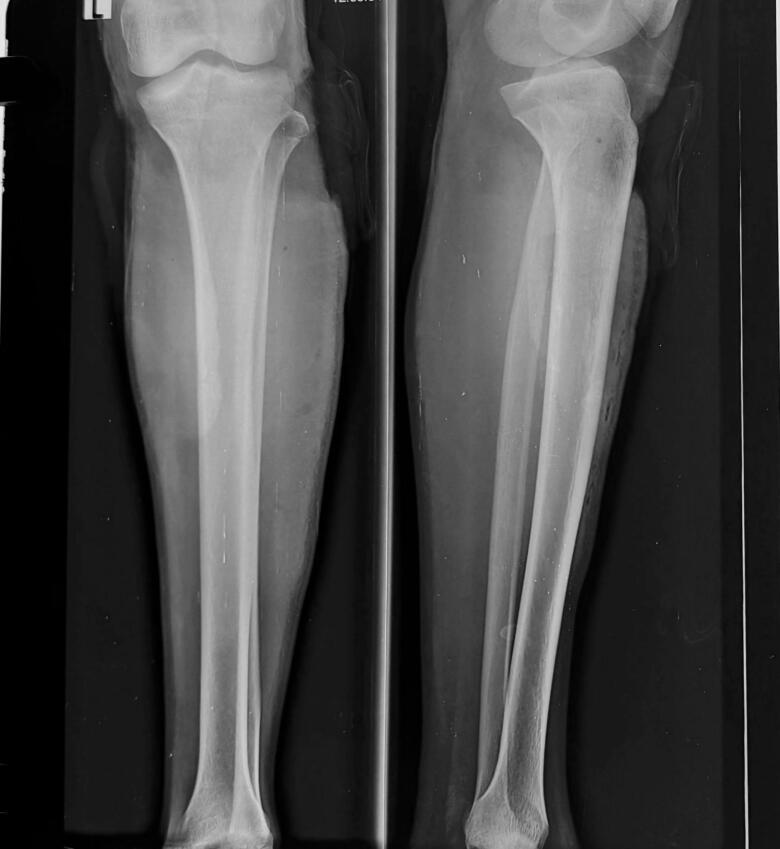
Pre-operative x-ray leg anteroposterior and lateral view shows no evidence of any fractures.
